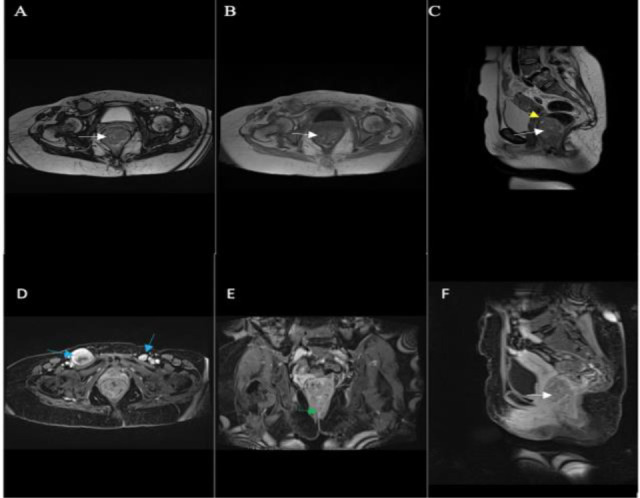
Magnetic resonance imaging (MRI) findings of anorectal mucosal melanoma of the patient. A-C; Axial T2 weighted; Axial T1 weighted; Sagittal T2 weighted; D-F Axial, coronal, and sagittal T1 weighted, fat-suppressed with contrast images, demonstrates a heterogeneously enhancing polypoid anorectal mass (white arrows) which shows prominent T2 hypointense and T1 hyperintense signal; it does not breach the rectal wall (yellow arrow). It extends to the anal verge (green arrow) with possible sphincteric muscle involvement and enlarged bilateral inguinal lymph nodes (blue arrows).
